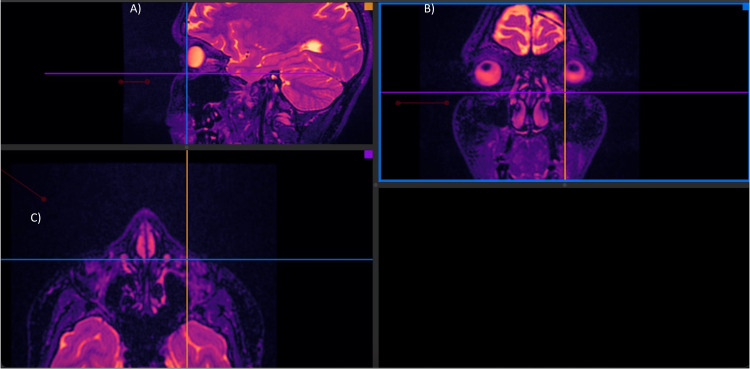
Facial MRI multiplanar reconstruction (MPR). These images can demonstrate in the three planes of space through a sagittal (A), coronal (B) and (C) axial view that Juvelook did not alter the signal intensity or radiological pattern of the MRI of the tissues after having been applied (two weeks post injection).
